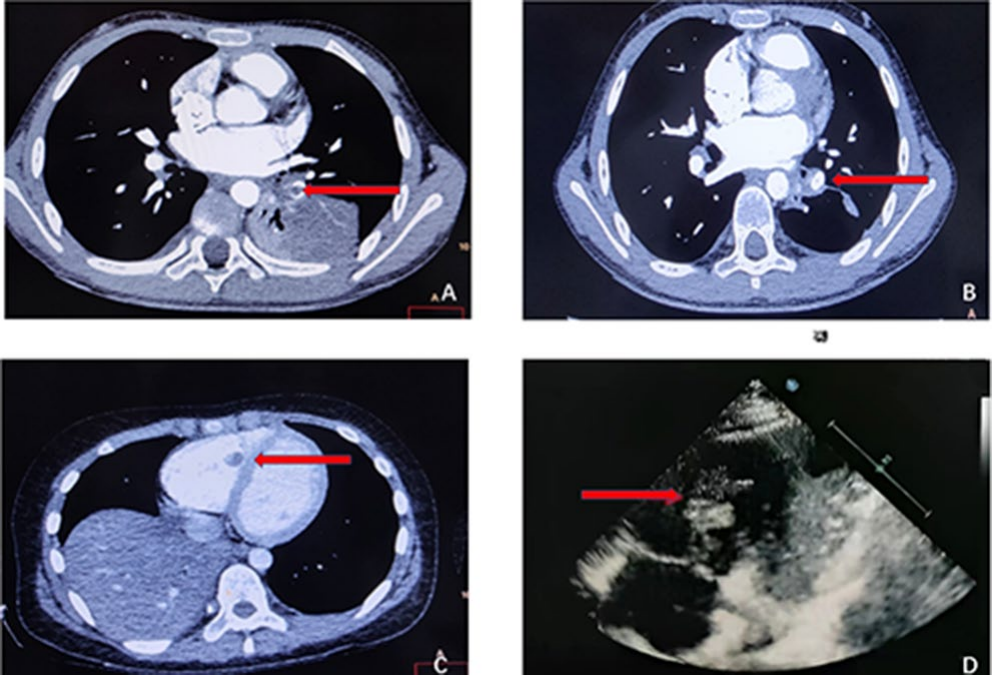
A: CTPA showed filling defects in the left pulmonary artery. B: Repeat imaging after anticoagulation shows disappearance of filling defect. C: CTPA showed filling defects in the right ventricle. D: Echocardiography showed mass-like echo in the right ventricle
